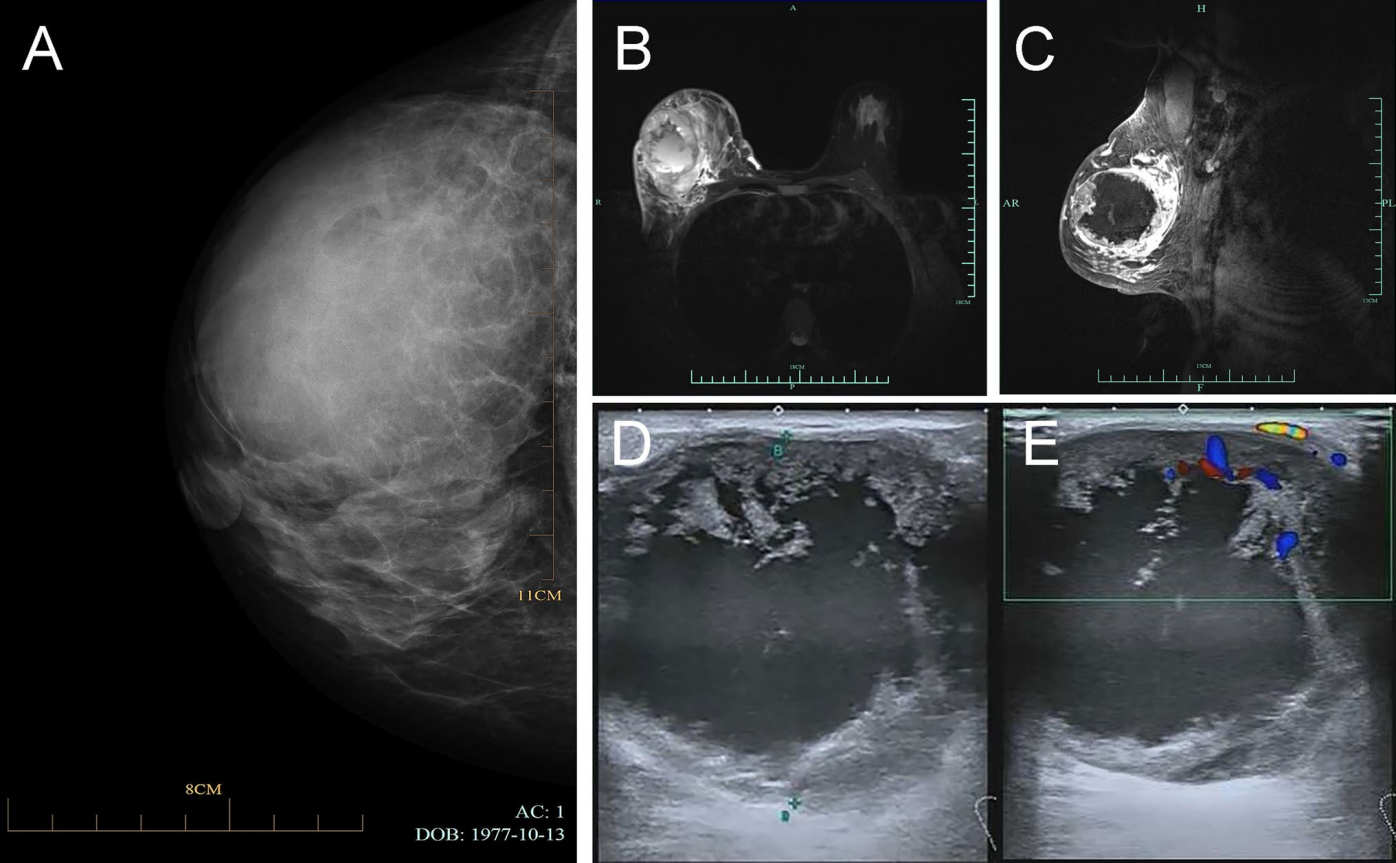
The imaging analysis of breast SCC in the upper outer quadrant of the right breast is presented. Mammography revealed a slightly elevated oval mass with a clear margin and homogeneous internal density A. The MR and DWI examinations (3.0 T) depicted a mass measuring 85 × 57 × 82 mm in size in the lateral quadrant of the right breast, invading surrounding tissues B, C. Ultrasonography revealed a 73 × 53 mm cystic-solid nodule with well-defined borders and a regular shape D, while blood flow signals were observed in the solid portion E
